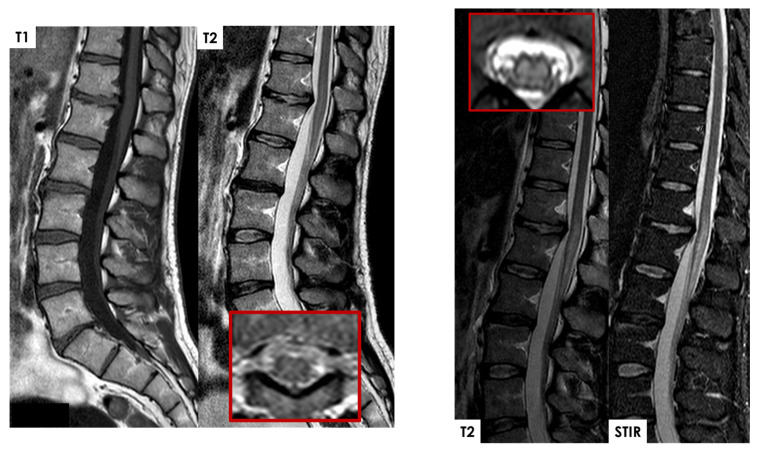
A 38-year-old patient in good health with moderate habitual physical activity. While performing gymnastics at home, the patient experienced the sudden onset of lumbar sensitive deficit, with rapid radiation to both lower limbs, associated with painful symptoms (described as cramp-like) in the same areas. This was followed by hyposthenia in both lower limbs, progressively worsening to the point of being unable to maintain an upright posture. Upon arrival at the emergency department, urinary retention was documented, and a catheter was placed. The patient underwent a CT angiography of the aorta, with a report showing no pathological findings, as well as a spinal MRI that was reported as normal (with a minimal osteophytic protrusion at the D10-D11 level extending posteriorly) (red lined figure). The following day, the patient had a repeat spinal MRI, which showed an intramedullary lesion hyperintense on T2W sequences at the D12-L1 level, with almost full axial extension (red lined figure), without bone alterations suggestive of trauma (DWI not available). The initial diagnosis was post-traumatic injury, followed by a suspicion of myelitis (subsequent diagnostic workup was negative for immune-mediated diseases). Outcome: ASIA D paraplegia from cord contusion at D12-L1 with motor level L4 on the right and L5 on the left, and sensory level L3 bilaterally. Neurogenic bowel and bladder. Most appropriate diagnostic hypothesis based on clinical and neuroradiological data: spinal ischemia. Spinal angiographic study (DSA) was performed after referral for a second opinion 8 months after the event: negative. The images on the right side of the slide are from the MRI performed 24 h after symptom onset.
